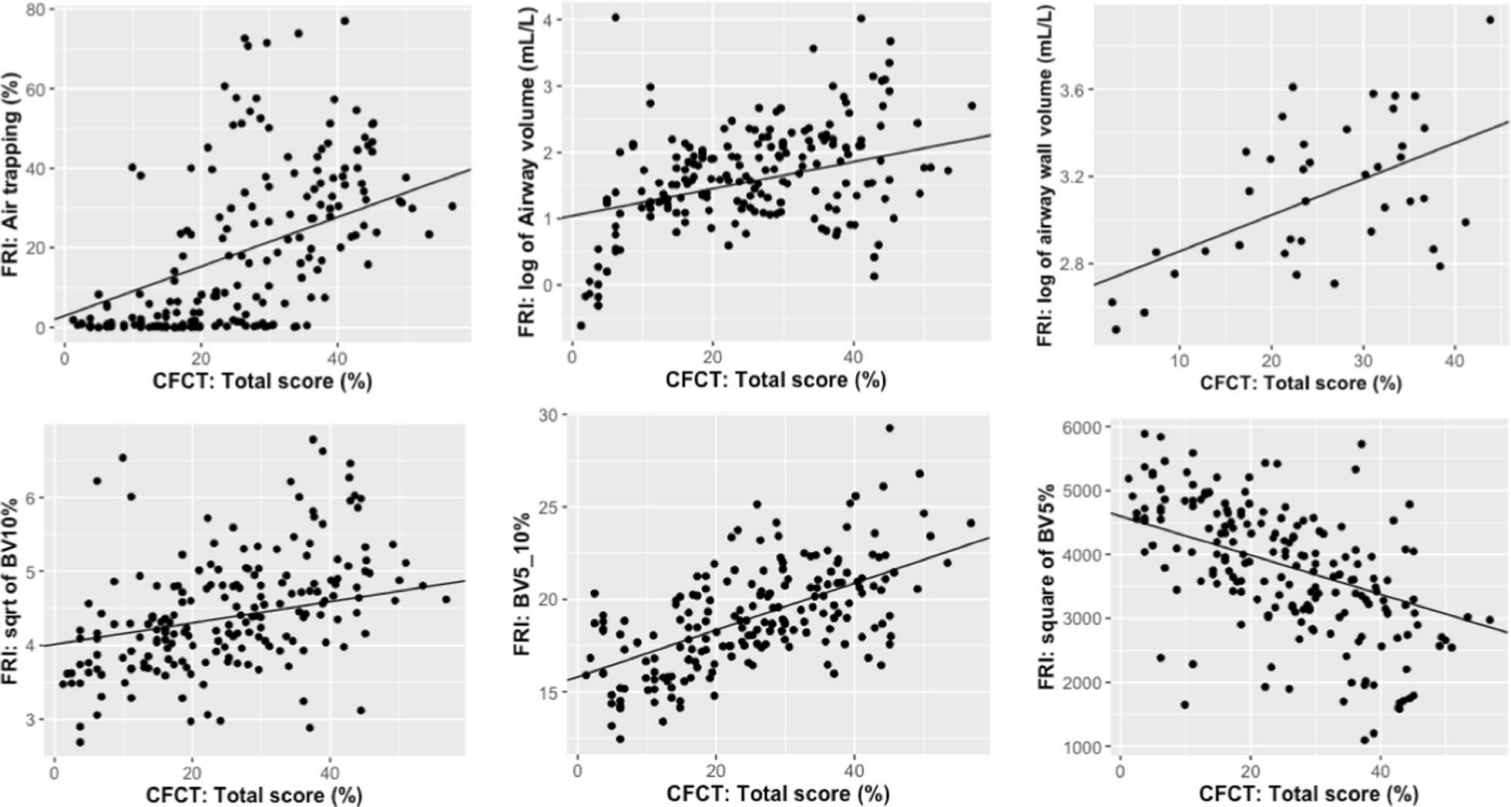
Association between FRI parameters and the CF-CT total score of all lobes with the regression lines of the linear mixed effects models
